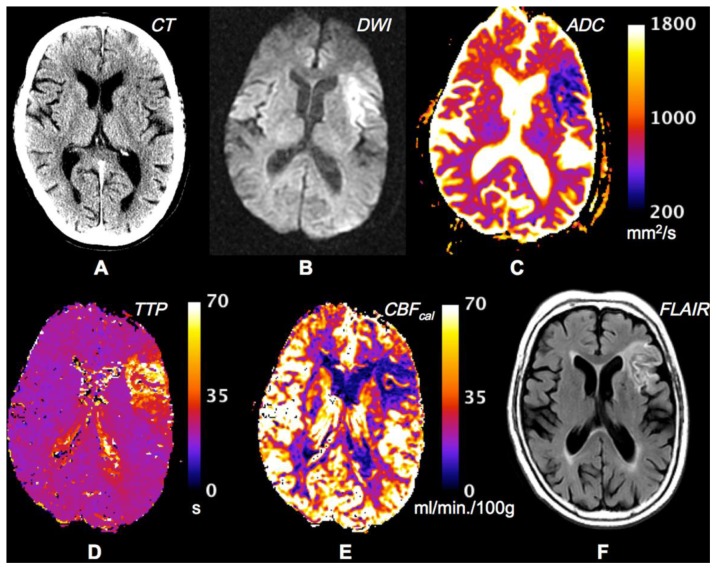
CT and MR imaging for an acute stroke patient (76-year-old female). (A) The CT image shows slight hypointensity in the left anterior insular ribbon, as indicated by the yellow arrow. The apparent loss of structural definition suggests ischemia in the middle cerebral artery (MCA) territory. (B) DWI image shows very hyperintense areas interpreted as a region of infarction. (C) ADC map shows decreased diffusion of water in absolute units within the markedly hyperintense DW region. (D) Time-to-peak (TTP) map calculated from a DSC perfusion sequence showing delayed flow to ischemic region. (E) Cerebral blood flow (CBF) map computed using deconvolution with an arterial input function as a reference, and then calibrated to white matter in the contralateral side to provide a semi-quantitative depiction of blood flow. (F) FLAIR image from day 30 follow-up MR exam shows final infarct to match closely with acute infarct. There was a delay time of 1.2 h from stroke onset to CT imaging, and an additional delay of 2.6 h to MR imaging. The patient was treated with intravenous tPA 1.5 h after onset–before the MR exam. DWI typically depicts infarction with greater lesion contrast as is the case here. The lack of a distinct perfusion-diffusion mismatch and no evident growth on follow- up may be at least partly attributable to recanalization occurring as a result of tPA administration (courtesy of Robert K Kosior, University of Calgary).
